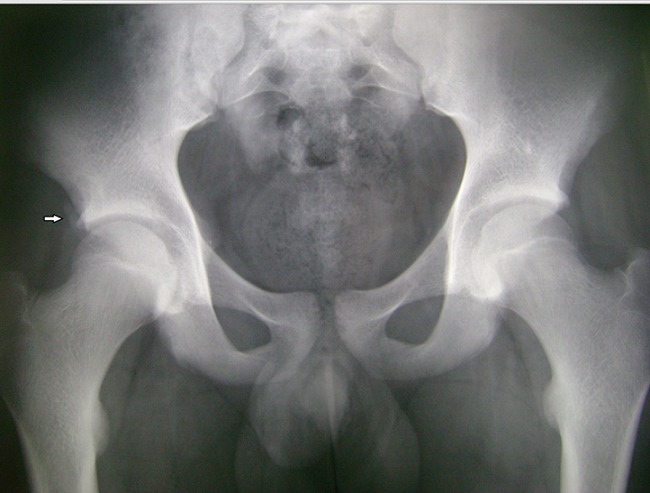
Radiographie du bassin face montrant un arrachement de l'EIAI
